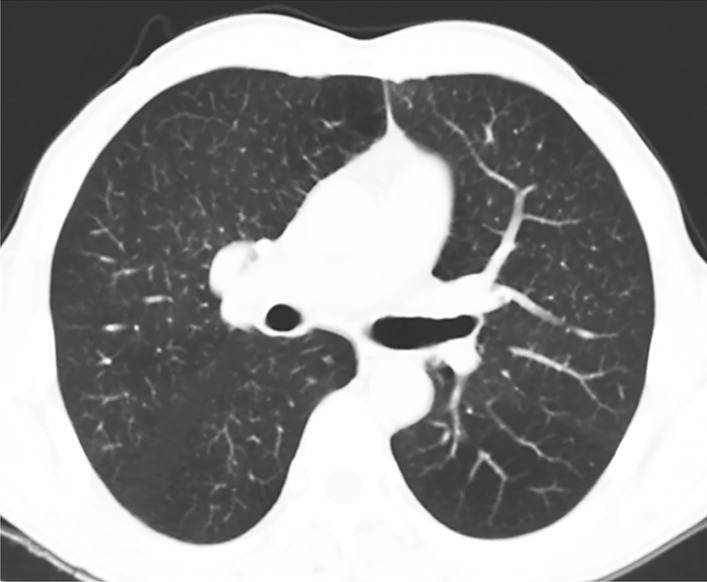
Chest CT scan showing no ground-glass exudation, patchy shadow, or consolidation in either lung before the incident
